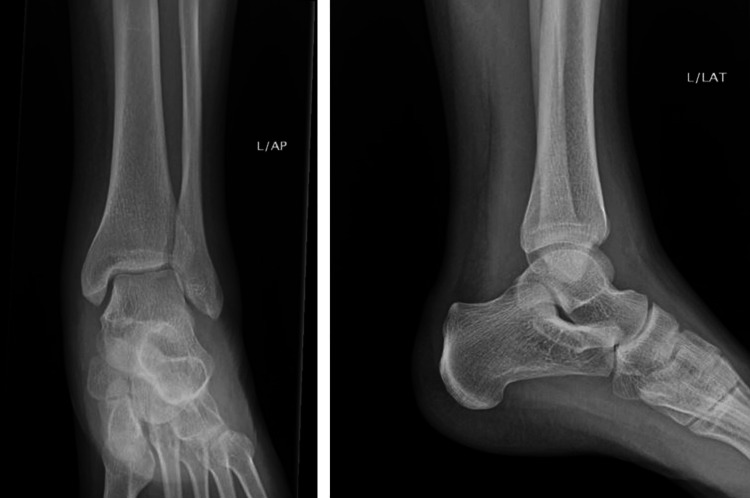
Left ankle X-rays anteroposterior (left) and lateral (right) Note that X-ray has a limited part in the diagnosis of septic arthritis, and further investigations are required. The diagnosis in this case remained clinical due to the rapid deterioration.
